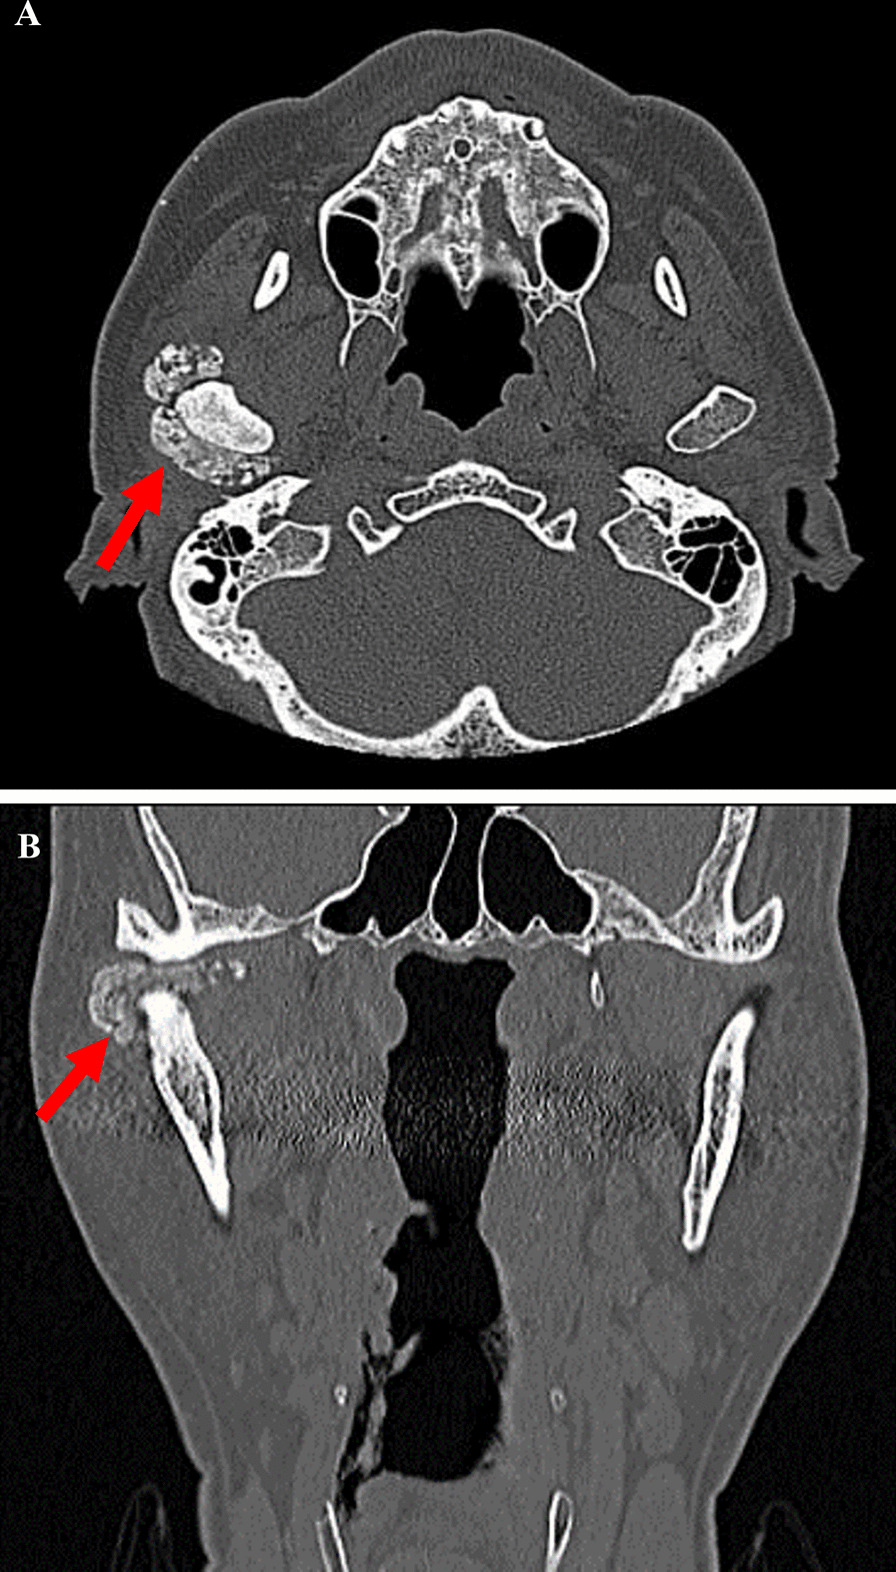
Preoperative CT images. A Axial CT image showing large intra-articular localized calcified lesions that abut the articular surface of the mandibular condyle of the Right TMJ. B Coronal CT image of the right TMJ indicated partial attachment of the calcified mass to the superior aspect of the mandibular condyle and cranial base
